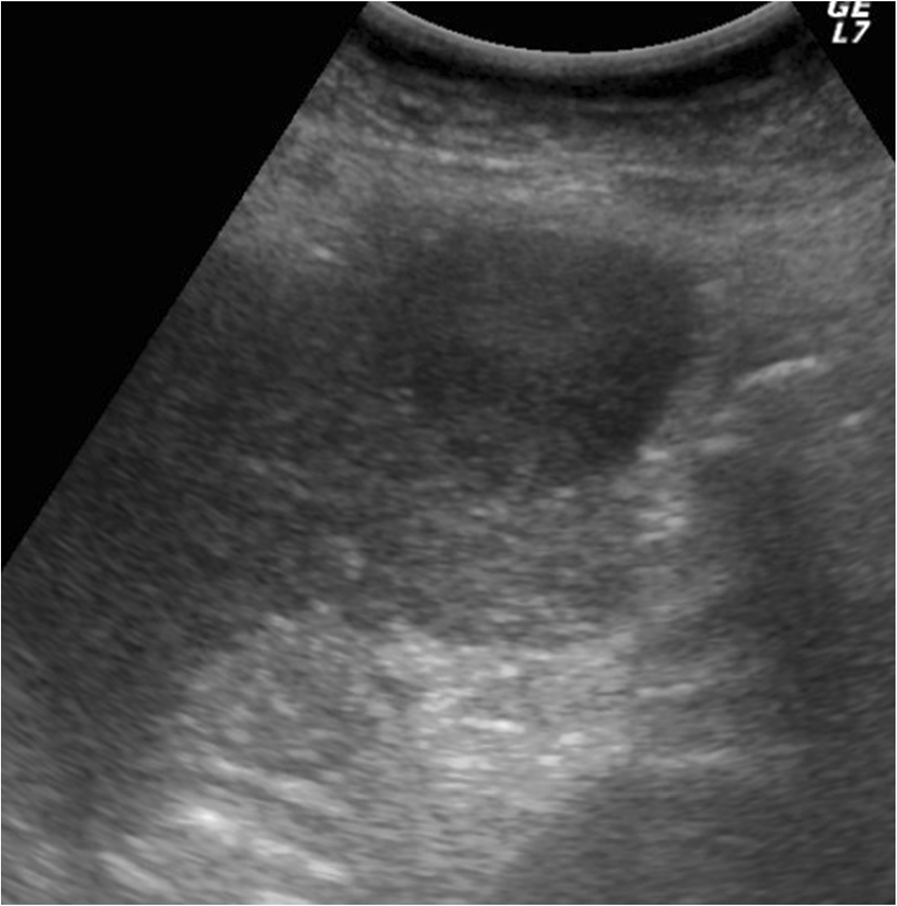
Preoperative ultrasonography. A hypoechoic lesion 34 mm in diameter was observed, and the appearance was not inconsistent with hepatocellular carcinoma
